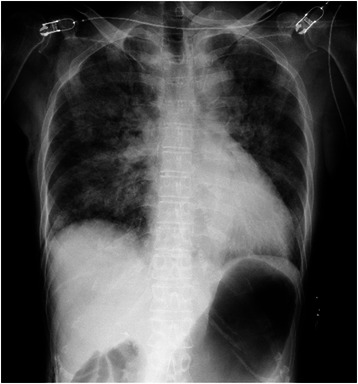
Portable chest radiograph. There were bilateral hilar pulmonary infiltrates, but the periphery of the lungs was relatively spared. Cardiac silhouette was not enlarged. There was air in the stomach.
